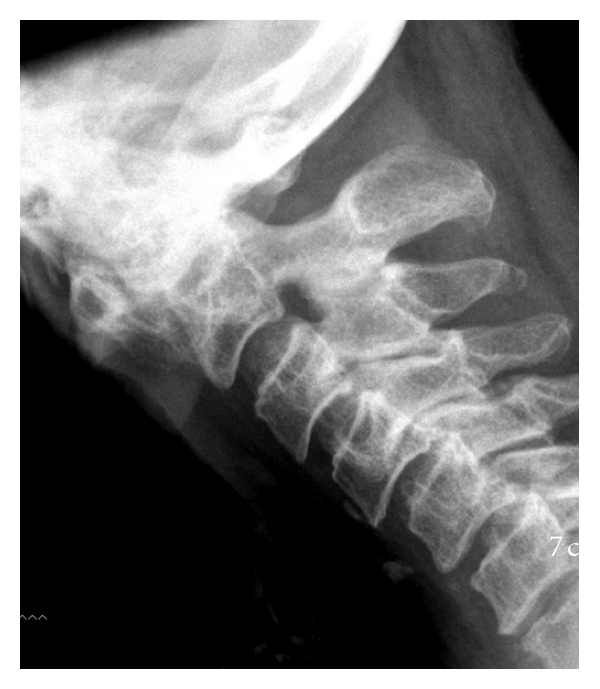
Lateral cervical radiograph of a patient demonstrating AA subluxation in flexion.
